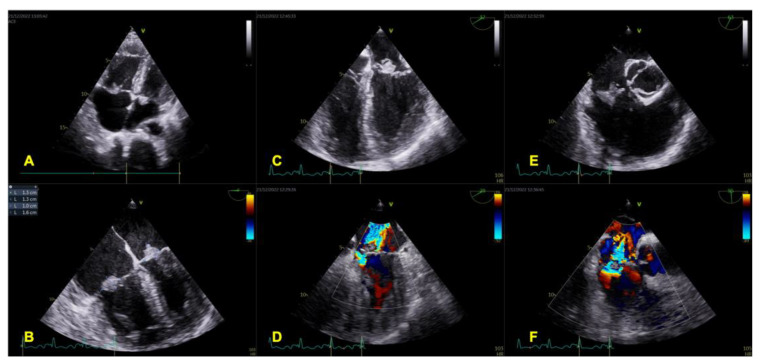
IE of both tricuspid and mitral valve. Vegetations are well-imaged by TTE, which is the first imaging tool in diagnosing IE (A). Their presence, size and location are confirmed by TOE (B,C,E). Both vegetations are associated with significant valve regurgitations (D,F). IE: infective endocarditis; TTE: transthoracic echocardiography; TOE: transesophageal echocardiography.
